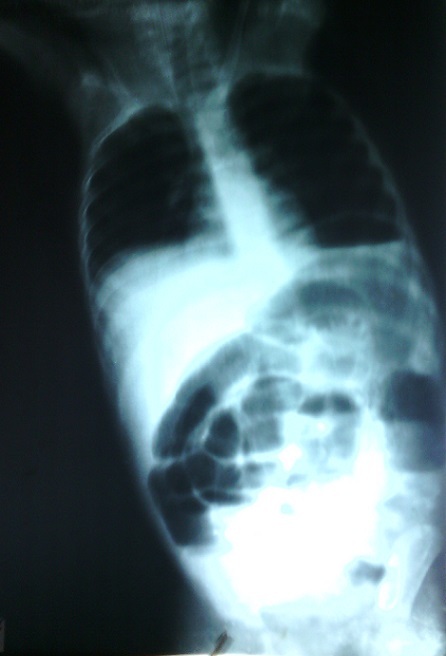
Radiographie de l'abdomen à blanc qui montre des niveaux hydroaériques centraux plus larges que haut et une dilatation importante du grêle rempli de gaz prédominant dans la fosse iliaque gauche, le flanc gauche, l'hypochondre gauche. Une opacité hypogastrique et dans la fosse iliaque droite
